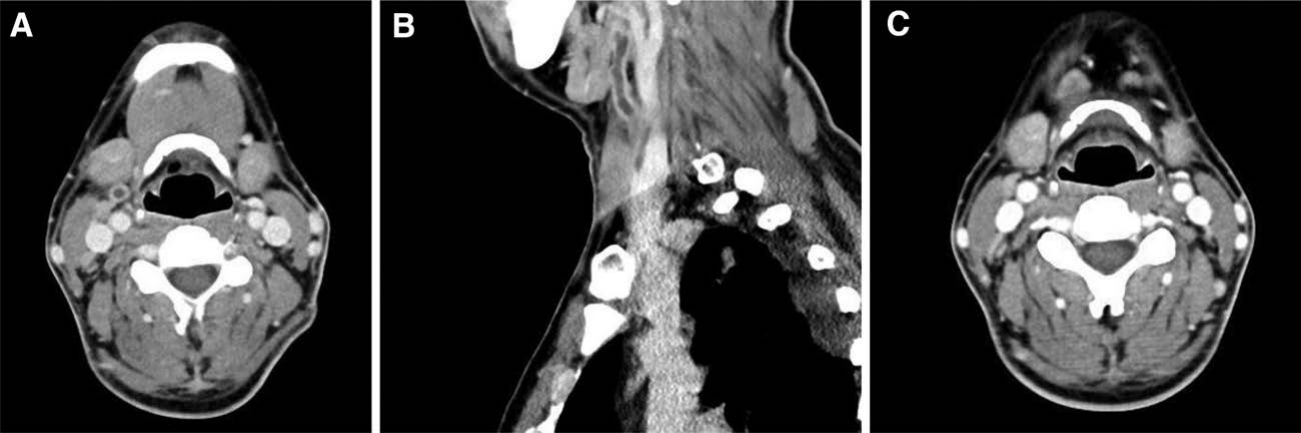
(A, B) Initial neck computed tomography showing a thrombus in the internal jugular vein. (C) Follow-up chest computed tomography on day 13. Neck computed tomography showing resolved thrombus.
